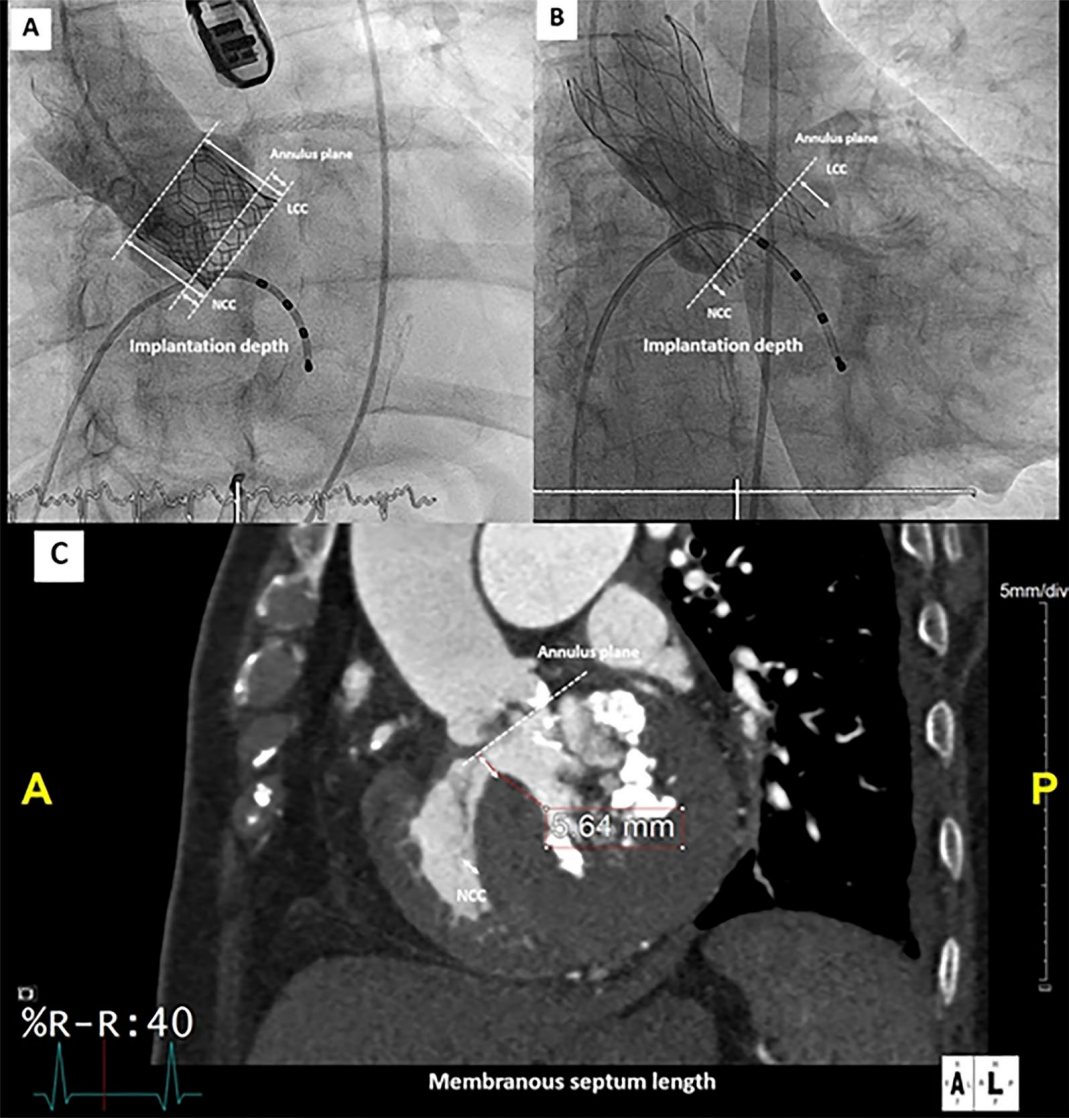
Example of angiography‐derived implantation depth (ID) measurement for balloon-expandable (A) and self-expanding (B) transcatheter heart valve (THV) as the range between the prosthesis below the aortic annulus plane (short arrows) at the noncoronary cusp to the native aortic annulus plane in the prosthesis in an orthogonal view. ID is expressed as length and percentage of frame height below the aortic annulus. Example of multislice computed tomography (MSCT)-derived coronal membranous septum (MS) length as the distance from the most superior thinnest part of the muscular interventricular septum to the annular plane in the coronal view under the systolic phase (at 40% of the RR interval) (C)
